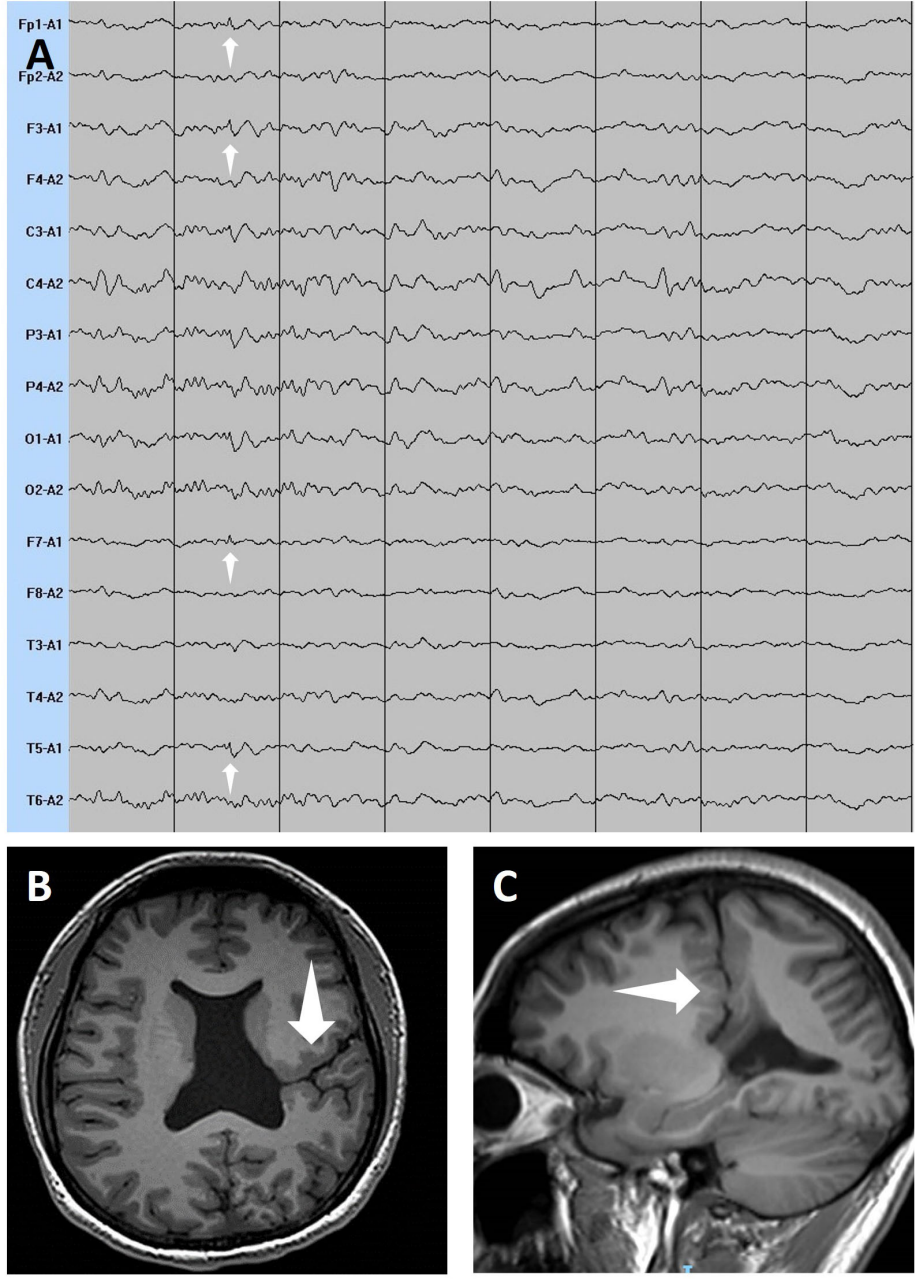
The white arrows in the electroencephalogram (A) show low to medium-amplitude spike waves in the left frontal pole, dorsolateral prefrontal cortex, and posterior temporal gyrus. The white arrows in the axial view (B) and sagittal view (C) of T1-weighted magnetic resonance imaging show a cleft extending from the subarachnoid space of the left frontal lobe region to the left lateral ventricle, filled with cerebrospinal fluid.
